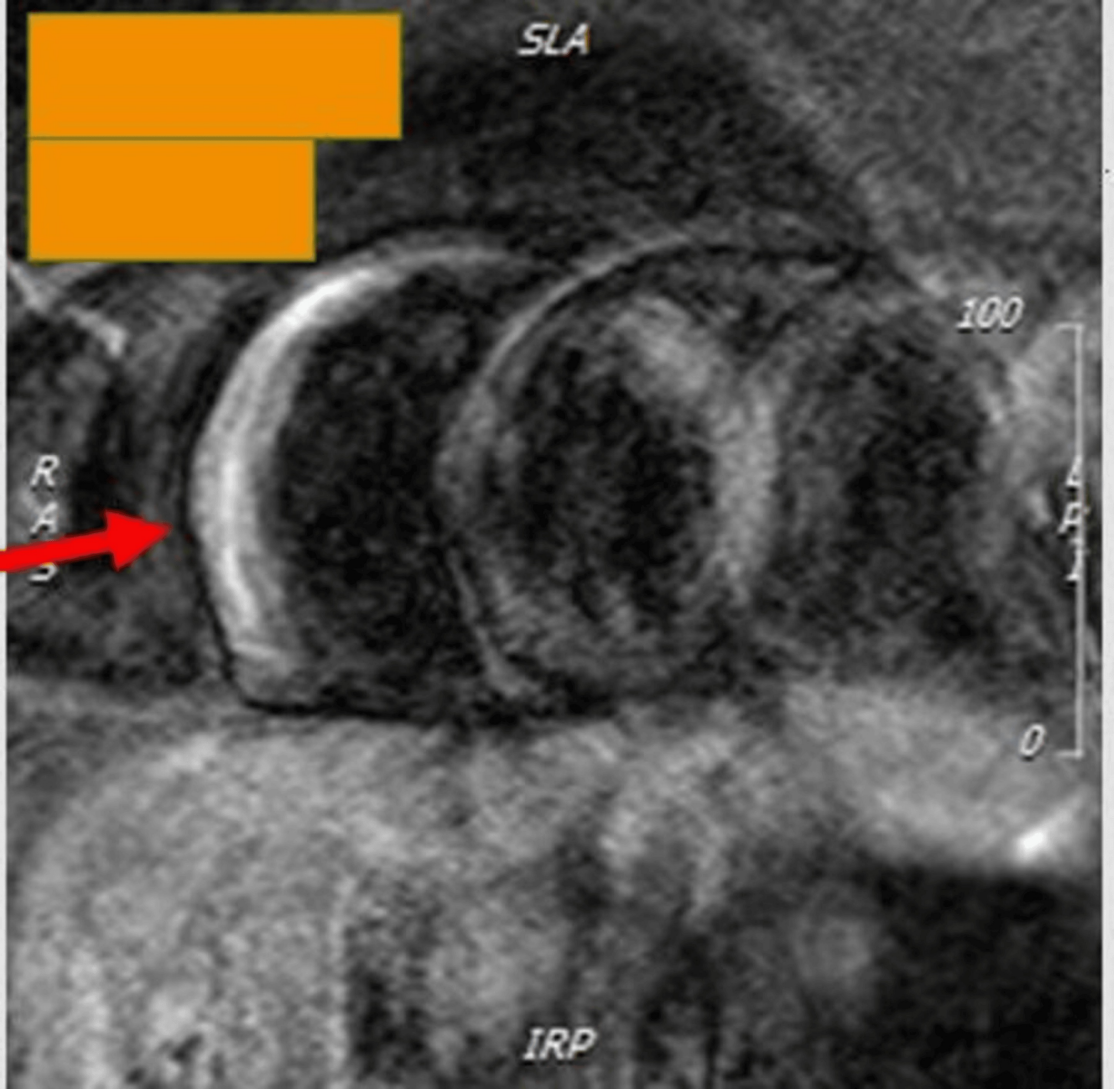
Cardiac MRI (2021). Note the myocardial edema.
